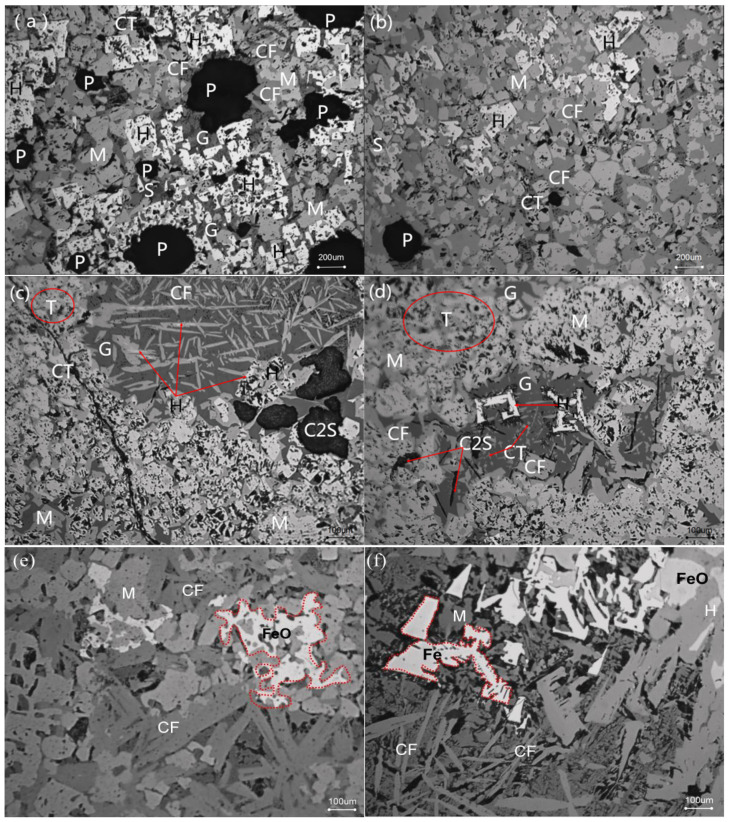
Mineralogy and microstructure of RVT sinter at different fuel levels (H: hematite, M: magnetite, CF: calcium ferrite, S: silicate, G: glass, CT: perovskite, P: pore, C2S: dicalcium silicate, FS: fayalite, T: over-melt zone): (a) Fuel-3.5%, (b) Fuel-4.0%, (c) Fuel-4.5%, (d) Fuel-5.0%, (e) Fuel-5.5%, (f) Fuel-6.0%.
